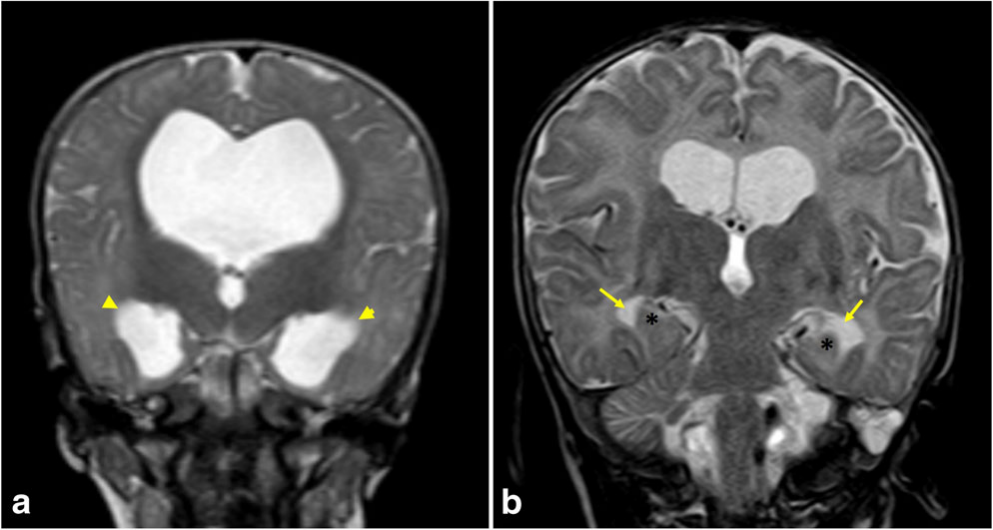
The value of coronal T2-WI in two different patients. a MRI of a 7-month-old girl with hydrocephalus showing commensurate dilatation of the temporal horns (arrowheads) with the lateral ventricles. b MRI of a 5-month-old boy with brain atrophy. The temporal horns (arrows) are small compared to the bodies of the lateral ventricles; they retain their normal shape and the hippocampi are not displaced medially (asterisk)
